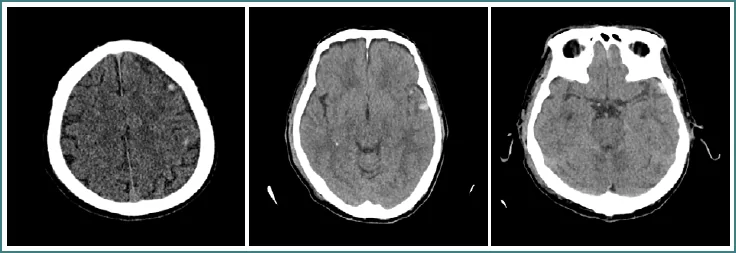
Brain CT on admission. Multiple parallel axial slices highlight the multifocal hemorrhagic contusions in the left frontal and temporal lobes.
